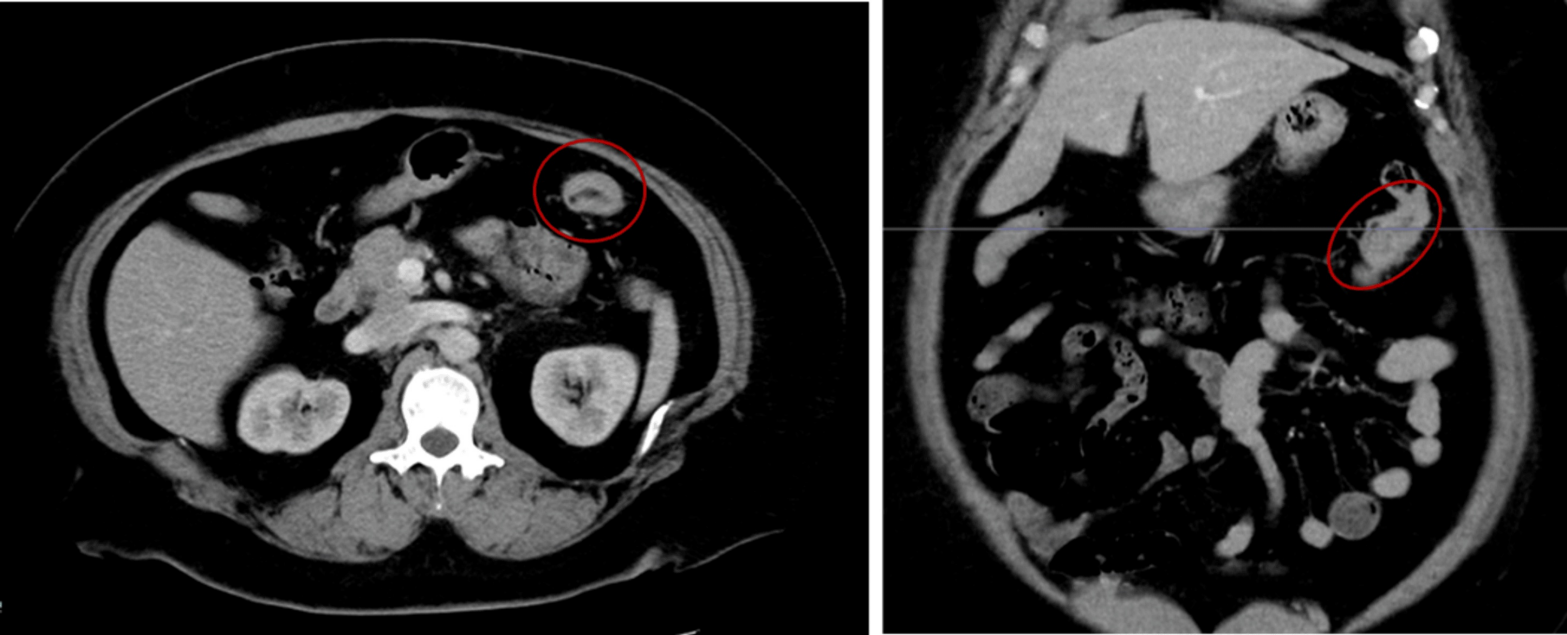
Preoperative CT CT shows transverse colon cancer in the splenic flexure (red-lined area) and no significant enlargement of the regional lymph nodes. CT: computed tomography
